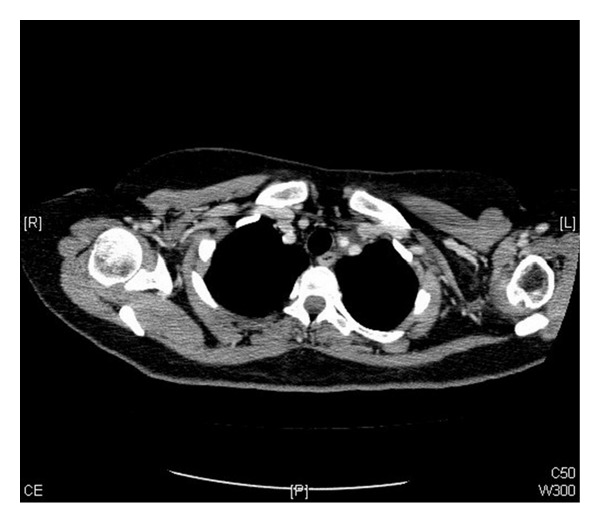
Postchemotherapy CT scan axial images showing a decrease in the size of the left supraclavicular nodes.
